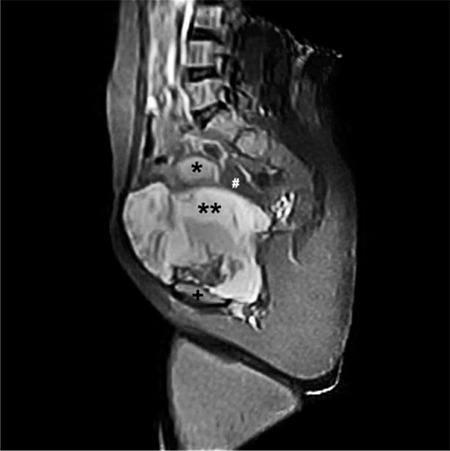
Magnetic resonance imaging showing a solid mass with 20x10 cm in diameter including calcified areas, and a mass consistent with myoma of 3.5 cm in diameter was observed in the cervico-isthmic region of the uterus
